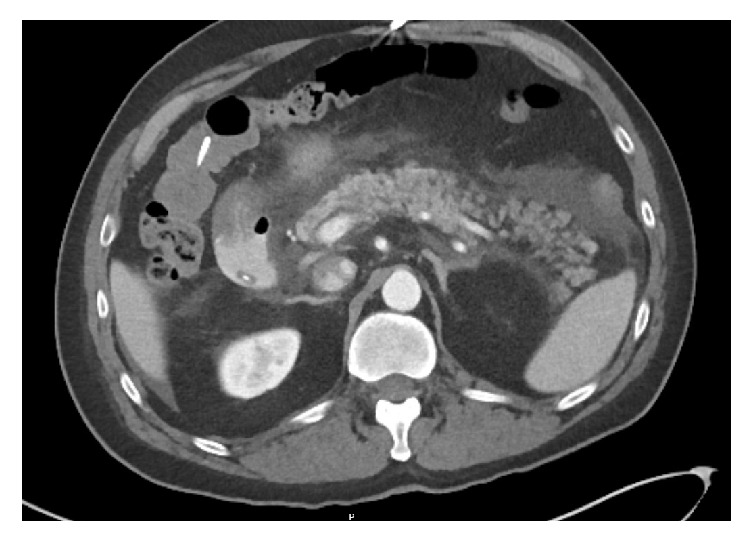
Abdominal contrast-enhanced computed tomography image. This image reveals diffuse edematous changes of the pancreas with surrounding inflammatory stranding and fluid consistent with acute pancreatitis.
